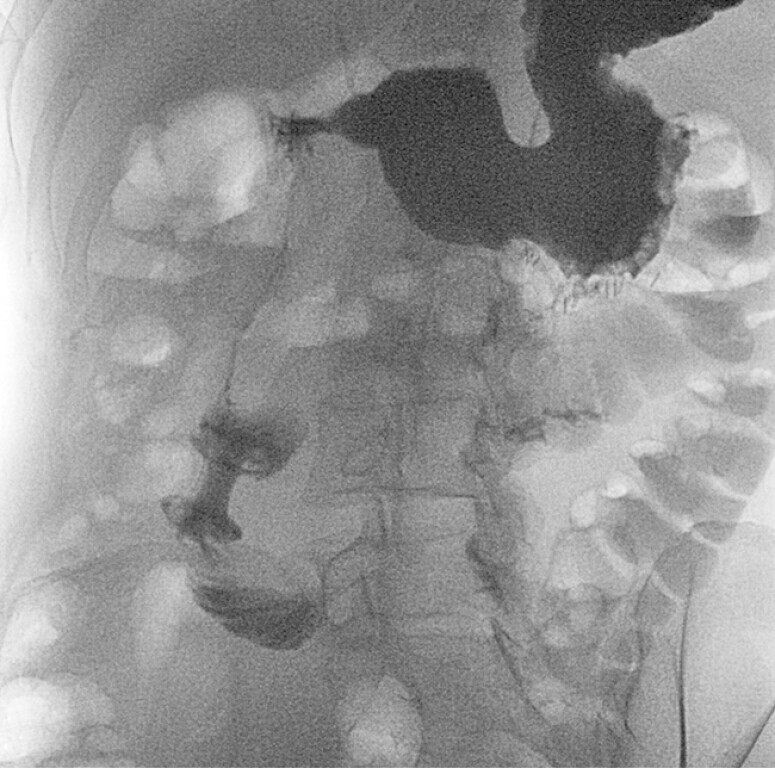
Upper gastrointestinal series performed 2 days after EUS-DJ demonstrating smooth contrast passage through the duodenojejunostomy. EUS-DJ, endoscopic ultrasound-guided duodenojejunostomy (EUS-DJ).
